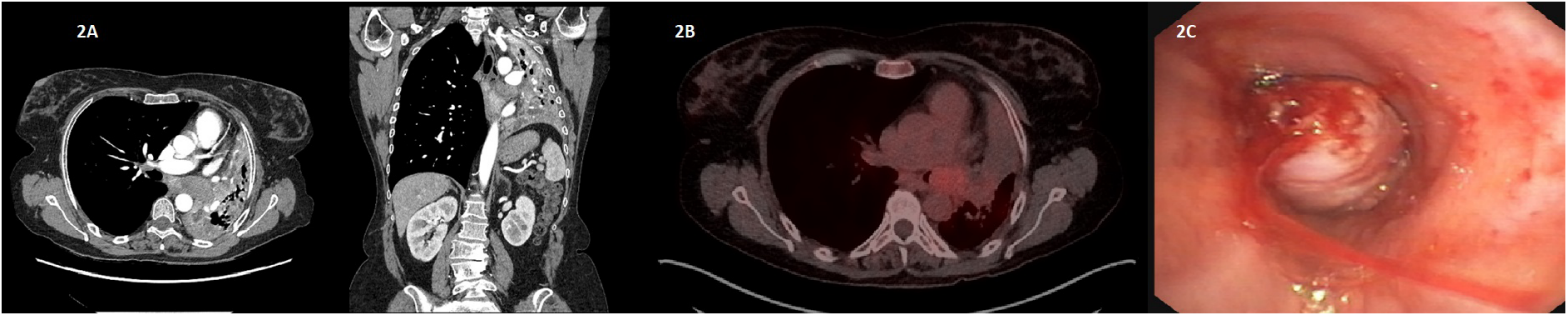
(A) Computarized tomography (CT) of the chest showing the endobronchial mass with associated atelectasis. (B) Axial representation of the FDG PET/CT of the patient showing the lung lesion with low tracer uptake. (C) Capture from the bronchoscopy test with the endobronchial lesion.
